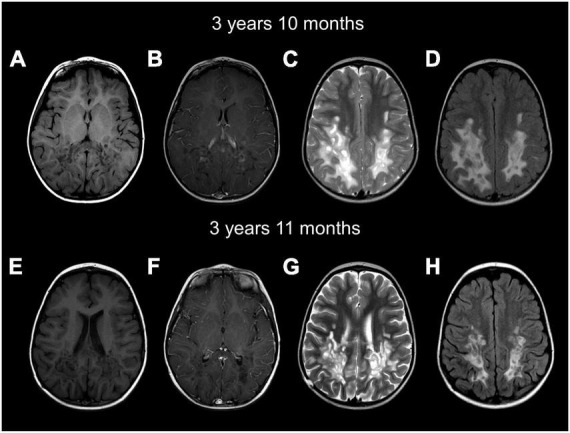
Brain MRIs at age 3 years and 10 months (A–D) and 3 years and 11 months (E–H) show a cavitating leukodystrophy with white matter abnormalities predominantly involving the posterior cerebral white matter and the splenium of the corpus callosum. Axial images show hypointense T1 (A,B,E,F) and hyperintense T2 (C,G) and FLAIR (D,H) parieto-occipital white matter. Numerous well-demarcated cysts are seen in the posterior white matter. Contrast imaging shows enhancement of the rim of cysts on the first MRI (B). FLAIR images show the white matter involvement, together with the numerous well-demarcated cysts (D,H). The follow-up MRI (E–H) shows mild improvement of the white matter abnormalities, less contrast enhancement and mild cerebral atrophy.
